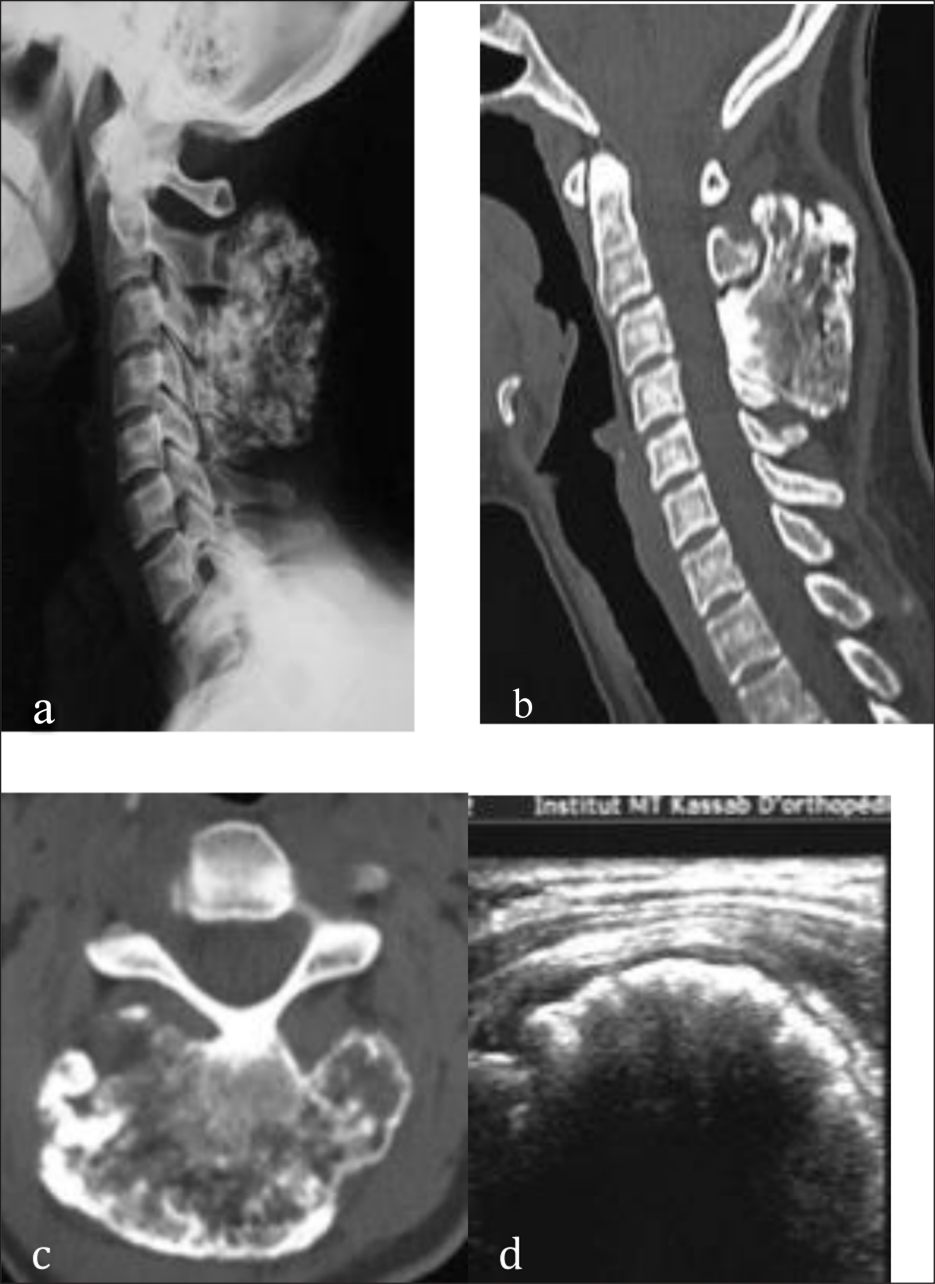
Osteochondroma of the cervical spine. Radiograph (a) shows an osseous mass located in the spinous processes of C3 and C4. CT scan (b, c) shows continuity of the osteochondroma with the spinous processes of C3 and C4. US (d) demonstrates a thin cartilaginous cap.
